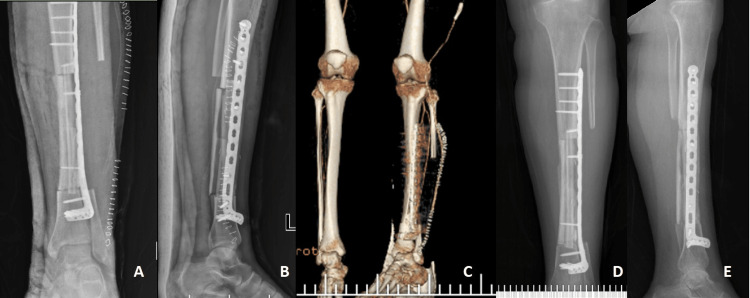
Postoperative images a) anterior-posterior radiograph, b) lateral direct radiograph, c) CT angiography found that the fibular artery was working, d) 12-month anterior-posterior radiograph, and e) 12-month lateral direct radiograph
